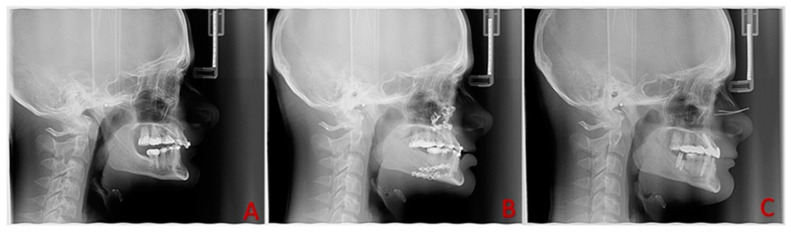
Lateral cephalometric radiographs, (A) preoperatively (T_0_), (B) at 2 weeks postoperatively (T_1_), and (C) at 9 months after the orthognathic surgery (T_2_). BTX-A injection into the ABDM seemed to prevent postoperative relapse. The SNA angle, SNB angle, ANB angle, mandibular length, and sagittal mandibular position were stable postoperatively. Courtesy of Dr. B.S.’s collection.
